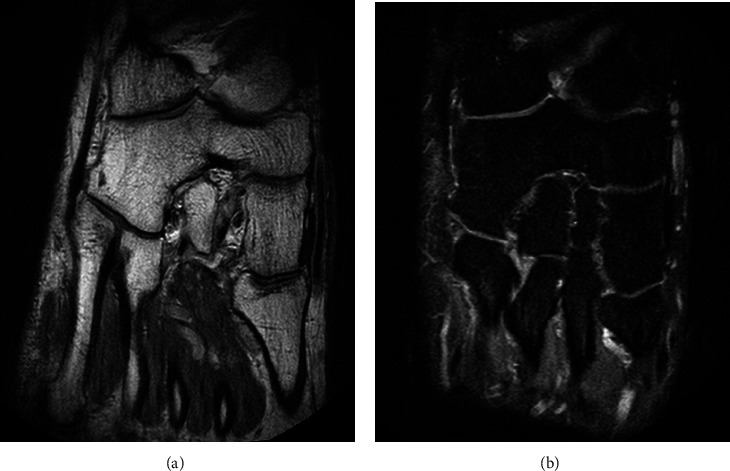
MRI of the right foot. Transversal T1-weighted (a) and PD-Fat Sat-weighted (b) images show a coalition between the cuboid and the navicular with a perfect continuity of the bone marrow and joint surfaces, without any bone oedema.
